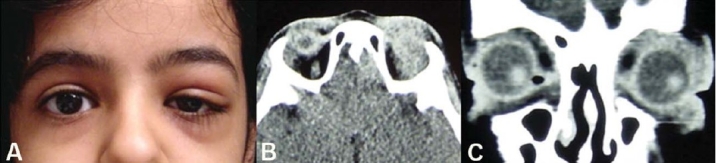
A 13 year girl presented with 1-month history of left upper eyelid swelling and pain (A). CT-scan (axial andcoronal) reveals inflammation of the left orbit (B and C).
